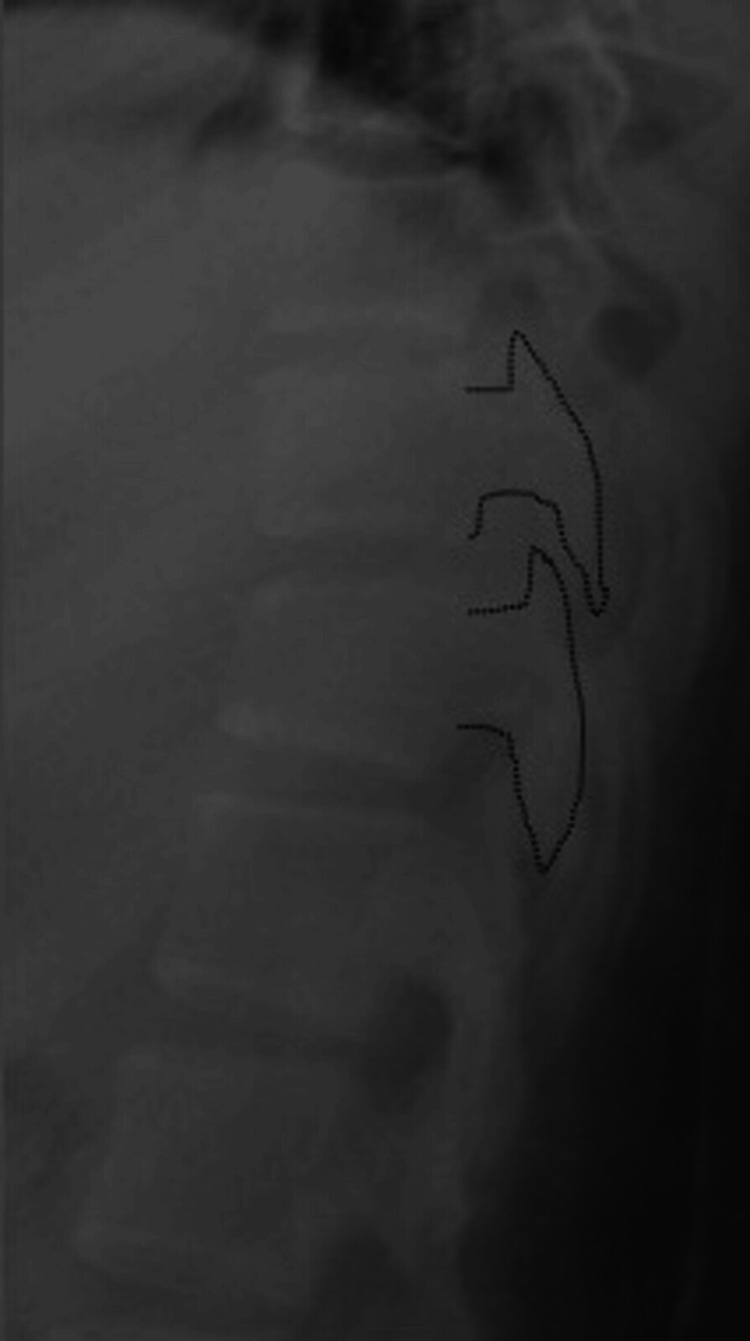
X-ray after closed reduction Reduction of facet joint dislocation achieved by hyperextension.
